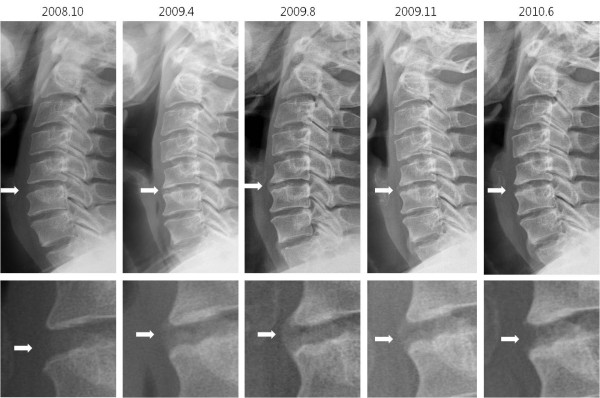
Cervical spine X-rays. Serial X-rays of the cervical spine revealed syndesmophyte formation between C5 and C6 and subsequent regression following peripheral blood stem cell transplantation in April 2008.
